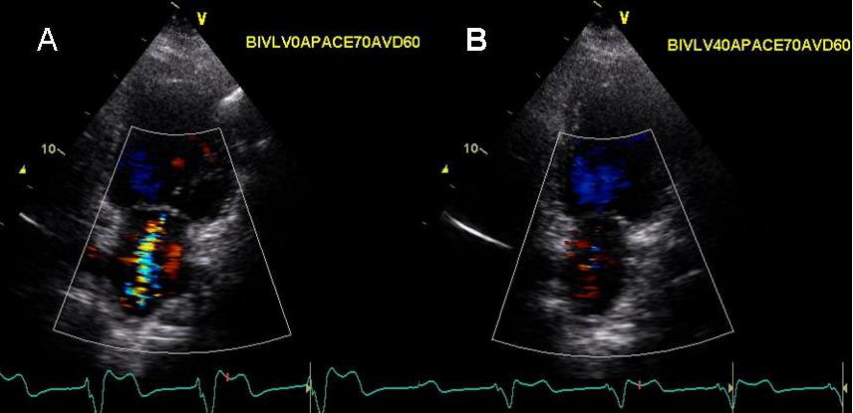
Color Doppler in 2 chamber view showing mitral regurgitation at baseline with VV delay of 0 ms (A) and with LV pre-excitation of 40 ms (B). A marked reduction in severity of mitral regurgitation (moderate MR decreasing to trace MR) is shown during LV pre-excitation.
